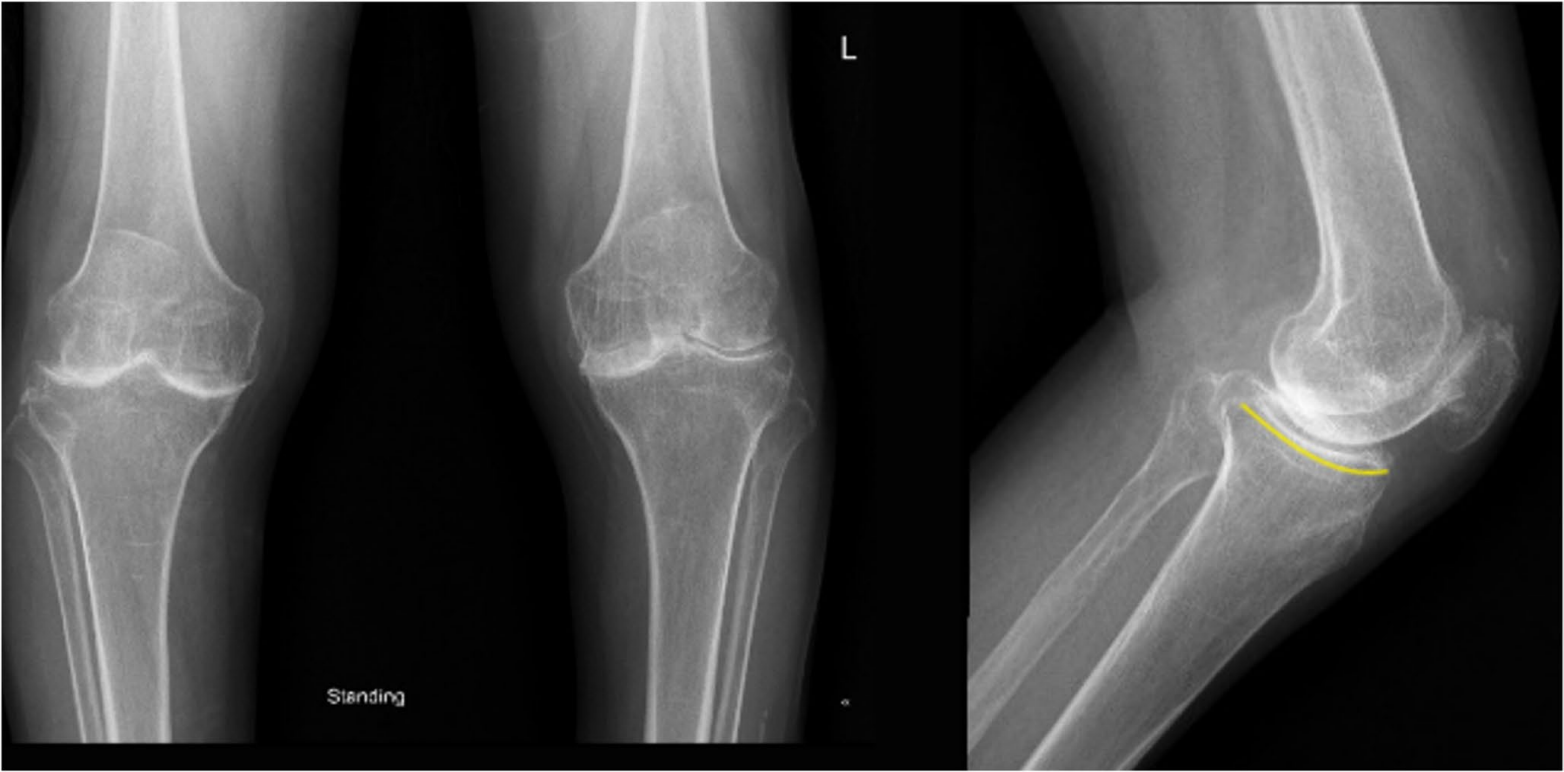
Bilateral knees AP radiograph in standing position shows severe degenerative change in his left knee with osteophytes and symmetrical reduced joint space with dished out proximal tibia articular surface (highighted line) and sclerosis noted over femoral condyles in AP view over bilateral knees
